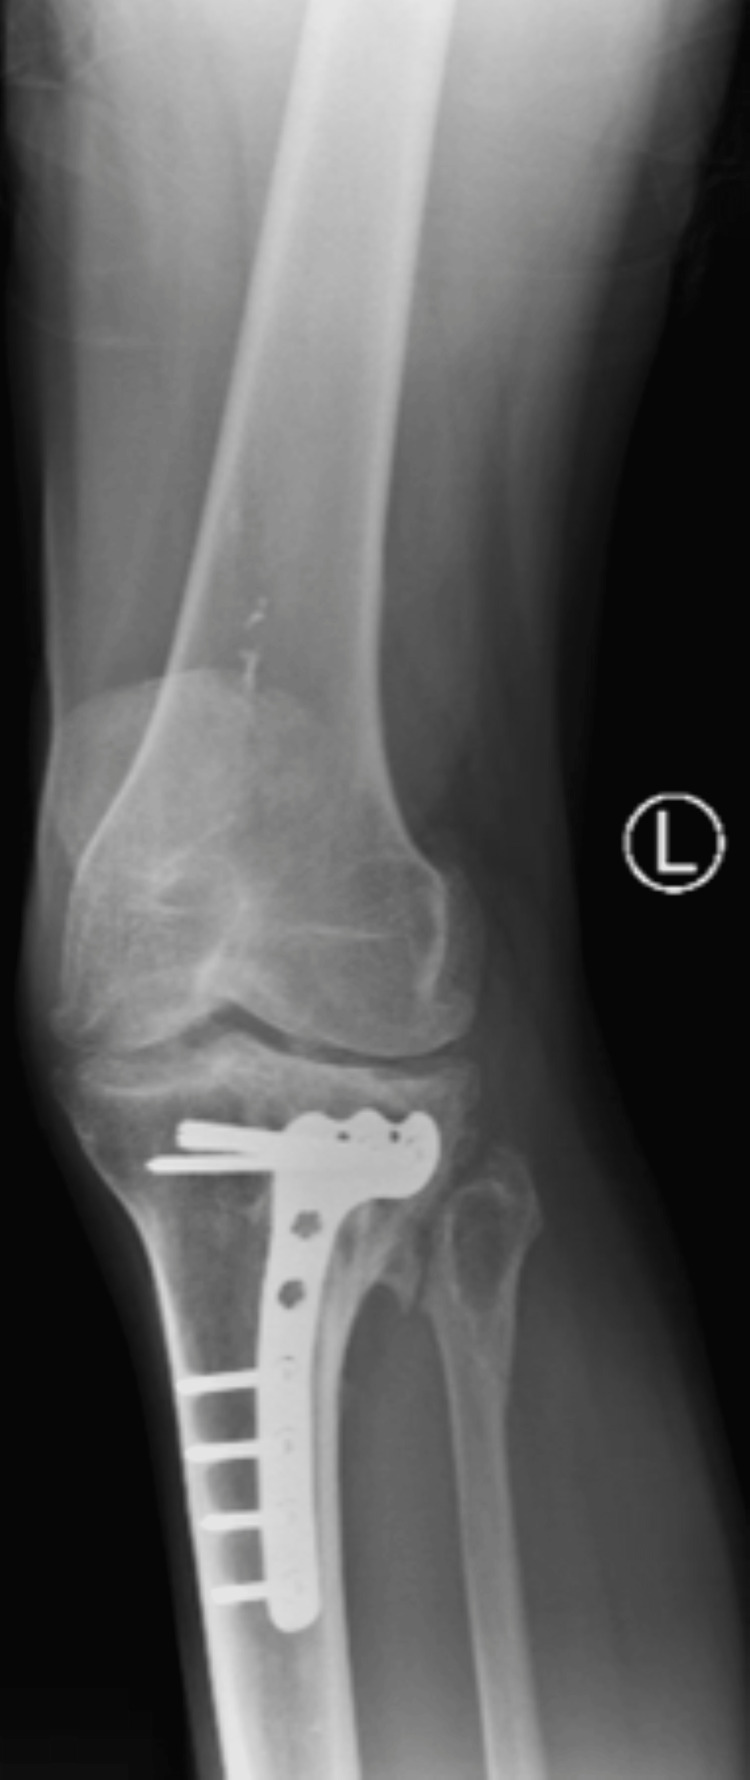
Preoperative radiograph of the left knee demonstrating retained lateral proximal tibial plate-and-screw fixation with advanced medial compartment degenerative changes.
